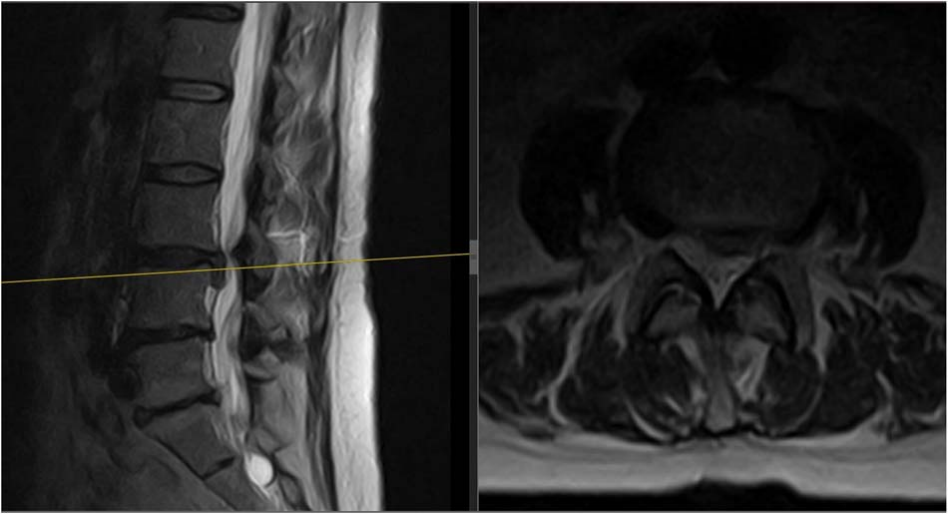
Initial MRI scan of the lumbar spine: initial MRI scan of the lumbar spine performed in July 2022 showing the left-sided L3/4 disc prolapse.
